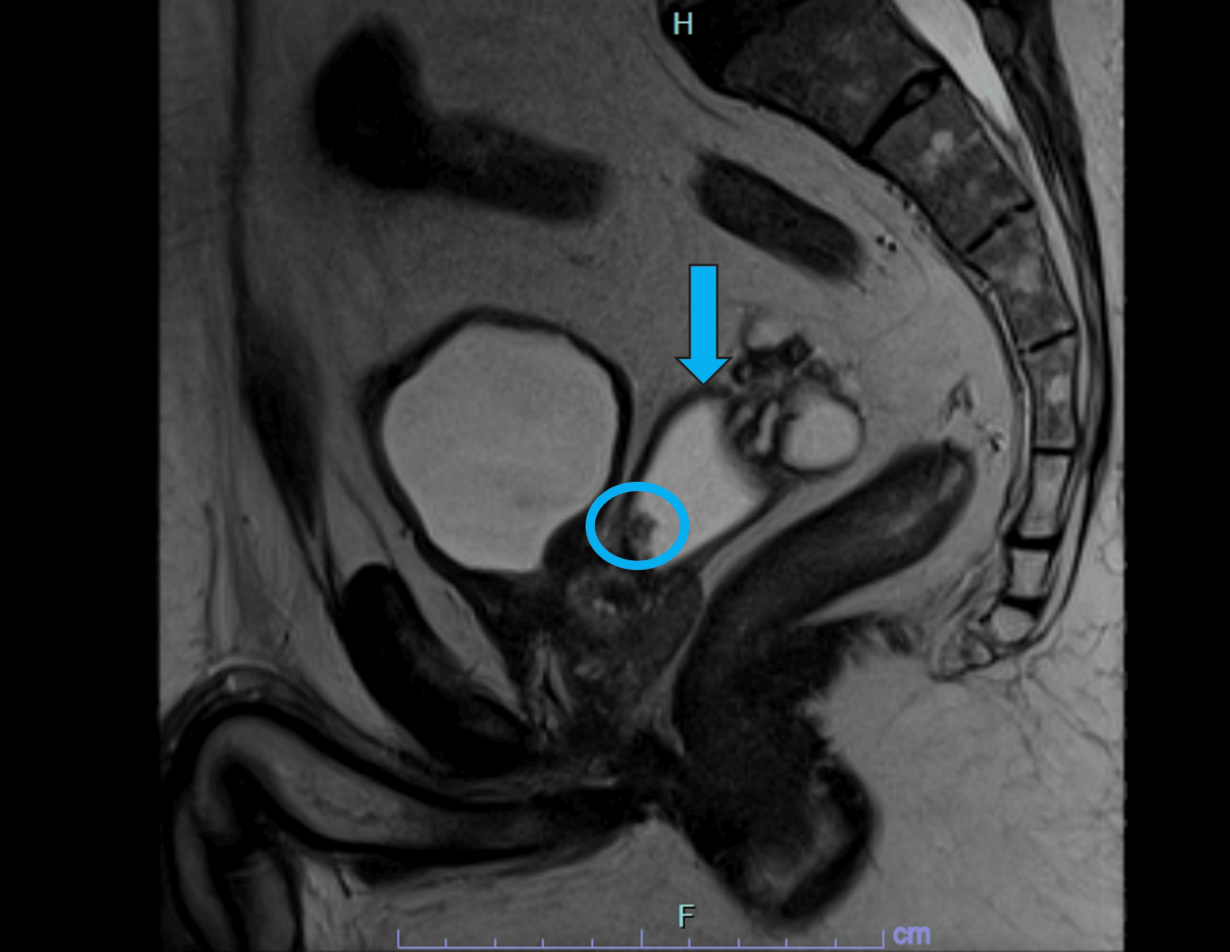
Sagittal T2-weighted (T2W) MRI of the pelvis demonstrating the ejaculatory duct cyst (blue arrow) with a small intralesional solid nodule (blue circle). MRI: magnetic resonance imaging
